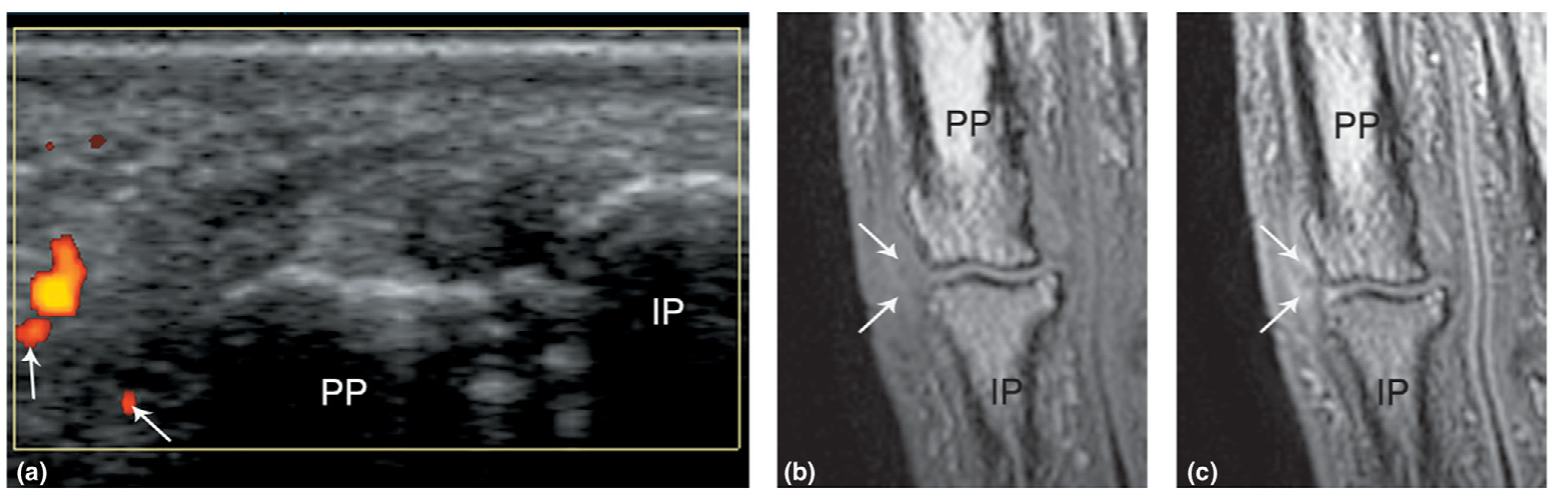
(a) Capsular/extracapsular changes (arrows) on power Doppler ultrasonography on the radial side of the 2nd proximal interphalangeal joint in a patient with psoriasis-associated arthritis. (b,c) The corresponding coronal T1-weighted magnetic resonance images before (b) and after (c) contrast administration showing capsular/extracapsular post-contrast enhancement. IP, intermediate phalanx; PP, proximal phalanx.
